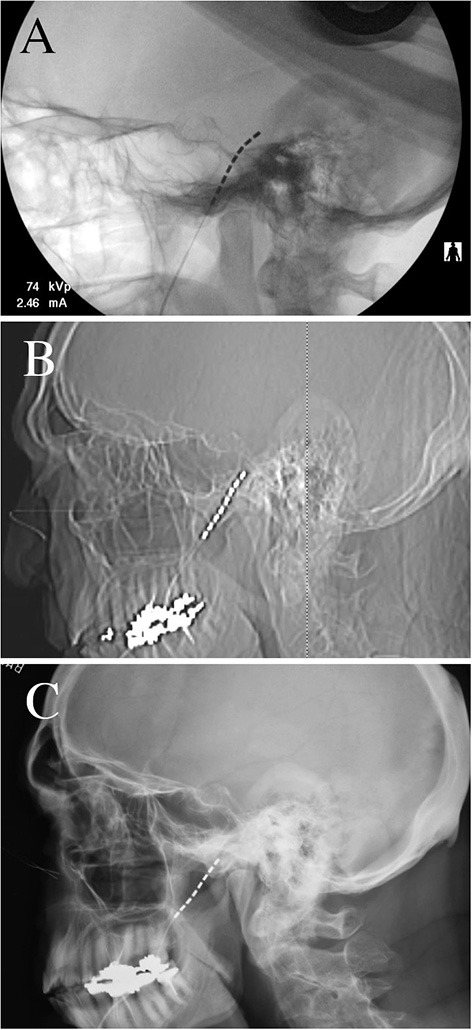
Radiographs of trial electrode placement. (A) Intra-operative lateral skull fluoroscopy image demonstrating placement of the electrode with the most distal contact within the foramen ovale. (B) Immediate postoperative lateral skull radiograph demonstrating that two-thirds of the electrode had migrated outwards (~35 mm) (C) 10-day postoperative lateral skull radiograph demonstrating complete migration of the trial electrode out of the foramen ovale, with no electrode contacts adjacent to the trigeminal ganglion.
